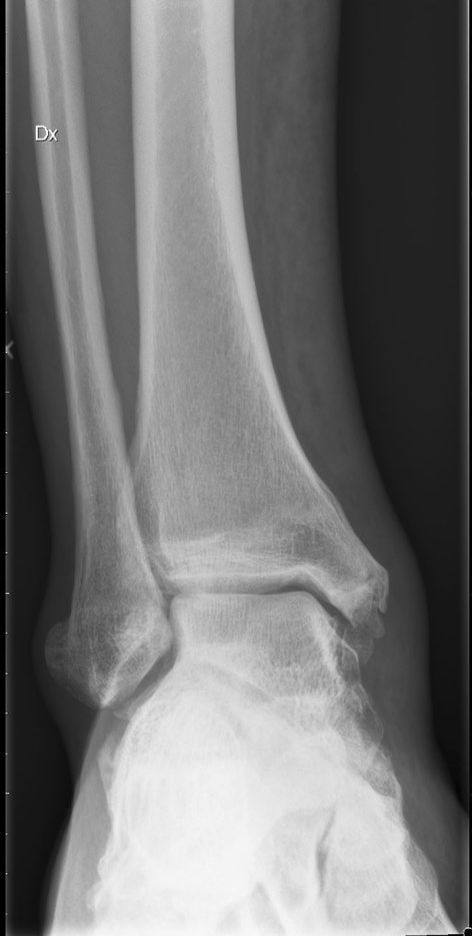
This X-ray image from the right leg and wrist from the patient presented in the case report is taken on 13th April
